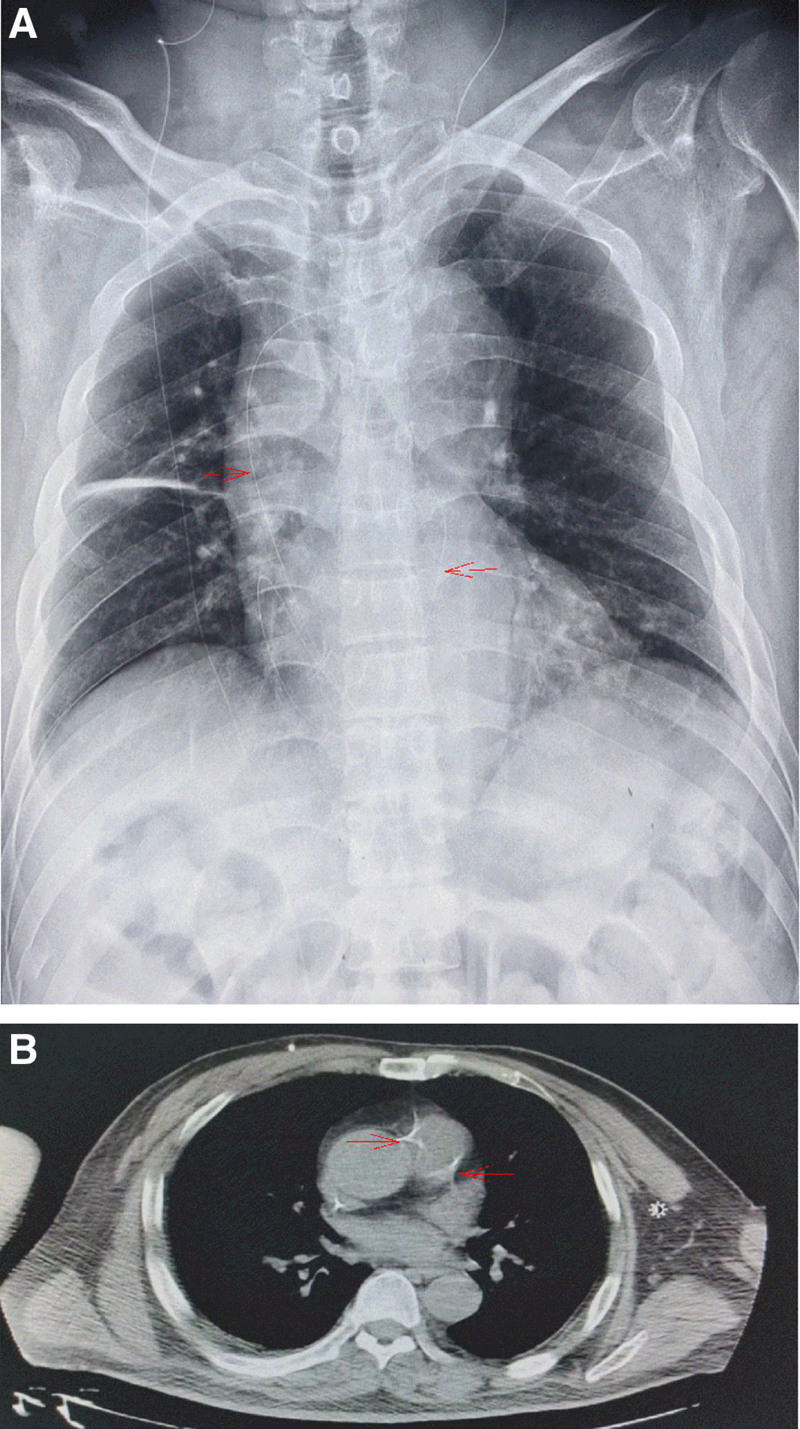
(A) Chest radiograph showing the left ventral end of the shunt coiled in the thoracic cavity (indicated by the red arrow). (B) Chest CT showing migration of the left ventral end shunt into the mediastinal cavity (indicated by the red arrow).
